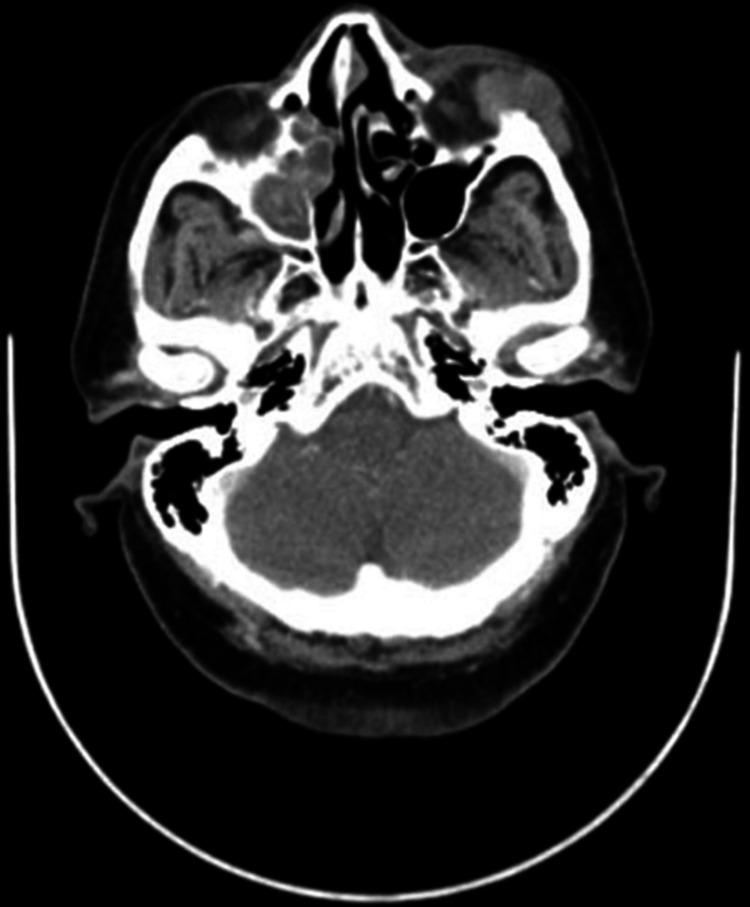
CT axial view of the head shows the subcutaneous mass overlying the left maxillary sinus
